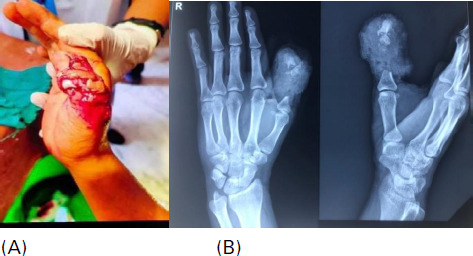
A) The middle third of the right thumb injury to distal to the metacarpophalangeal joint, B) Plain radiograph of thumb at the time of presentation.
